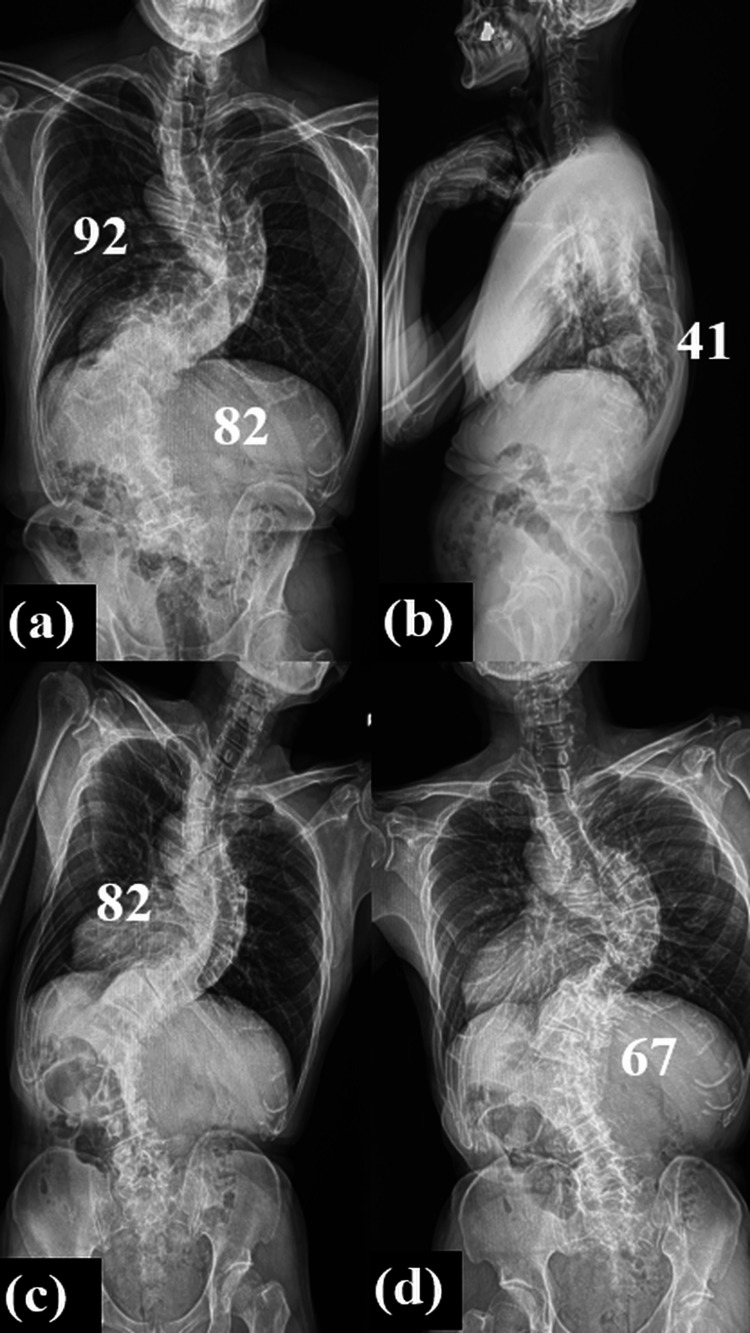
Preoperative radiographs Preoperative (a, b) standing posteroanterior radiographs showing the Cobb angles of the (a) thoracic and lumbar curves (92° and 82°, respectively) and (b) thoracolumbar kyphosis (41°) and (c, d) side-bending radiographs showing loss of flexibility.
